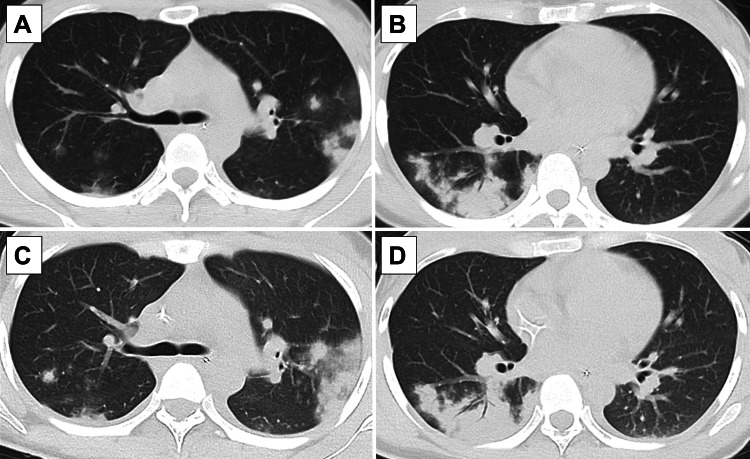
Chest computed tomography Computed tomography images reveal diffuse nodular opacities in both the lungs and infiltrative shadows with bronchial translucency in the right lower lobe. Some solid nodules display ring-shaped ground-glass opacities at their margins. Rapid progression of the infiltrative shadows was observed within a few hours (A, B: during oxygen desaturation on day 2 of hospitalization; C, D: after ICU admission).
